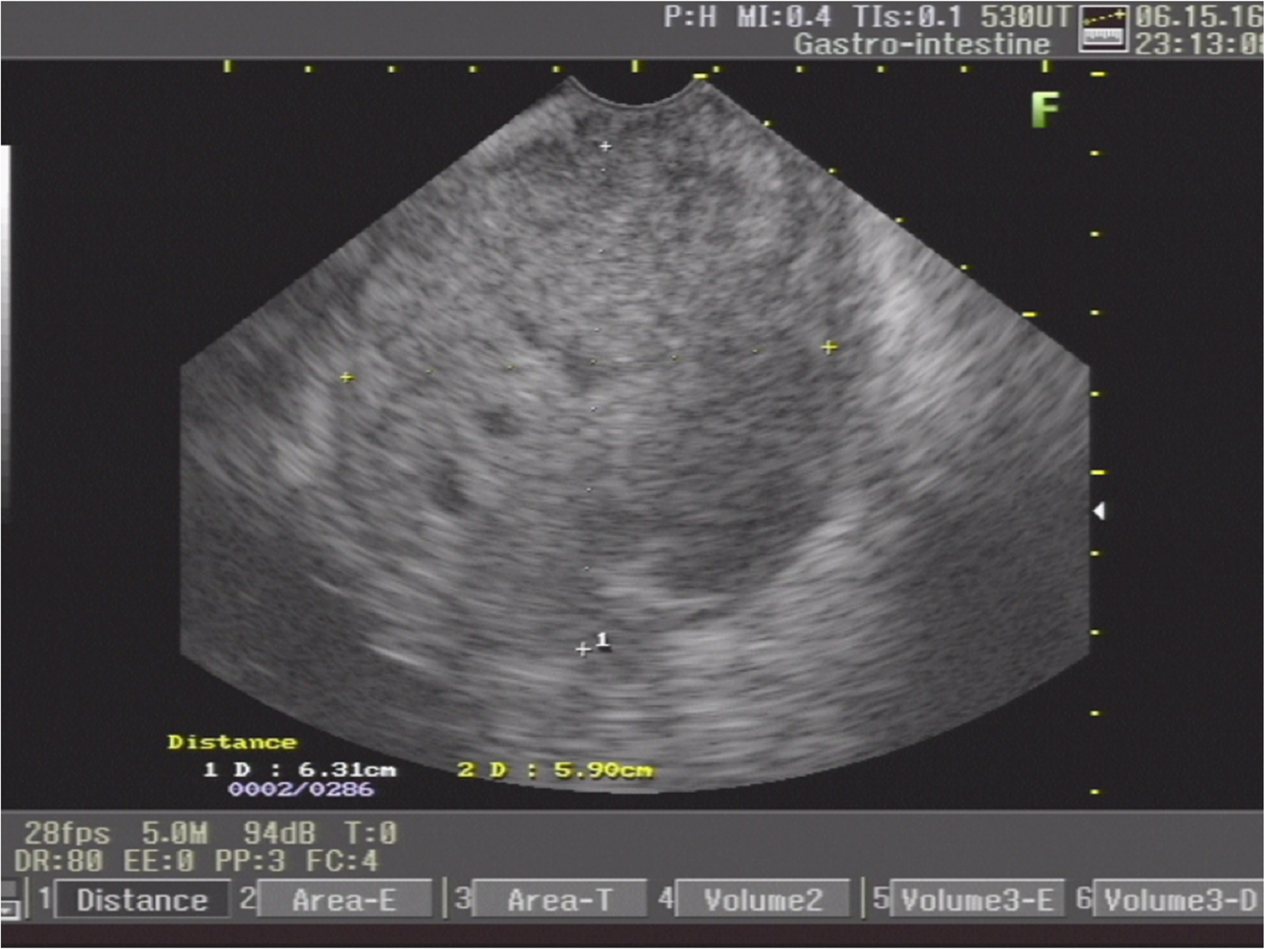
Endoscopic ultrasound showing a homogeneous hypoechoic mass with areas of necrosis as a rectal subepithelial lesion originating at the fourth layer (muscularis propria)
